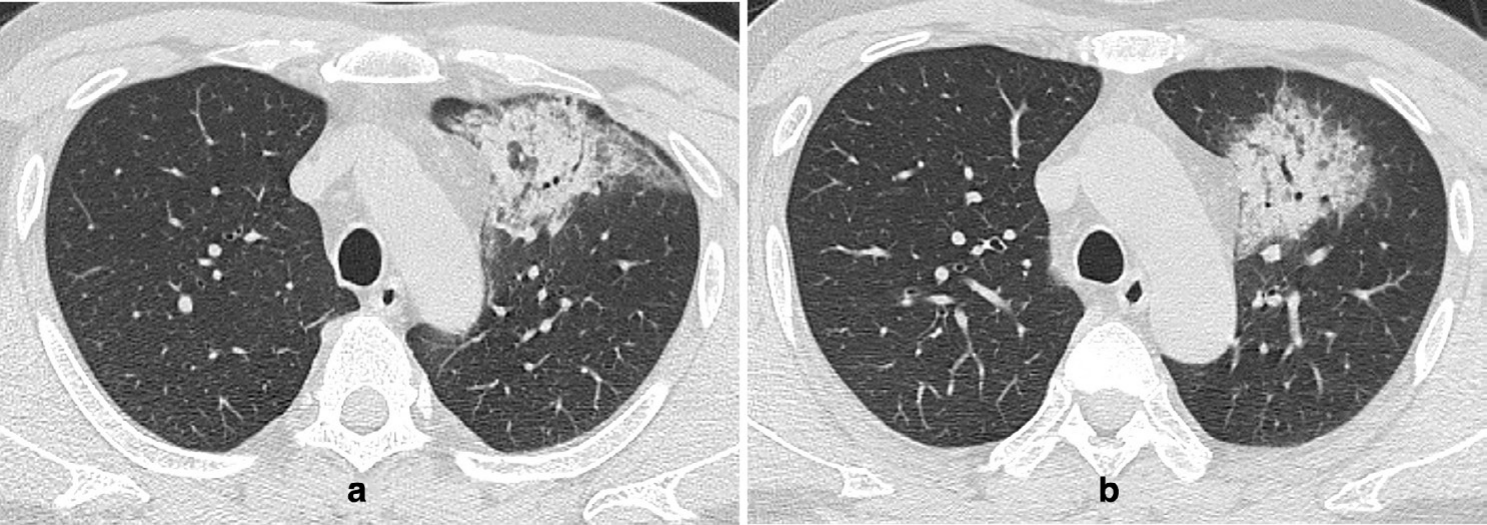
(a-b) An axial CT image obtained without intravenous contrast in a 43-year-old male showed peripheral/subpleural patchy consolidation in the anterior of the left upper lobe, with interlobular septal thickening, air bronchogram and linear opacities. He had a history of living in Xiaogan, Hubei Province and presented with fever for 1 week. Nasopharyngeal swabs for SARS-CoV-2 RNA were positive.
